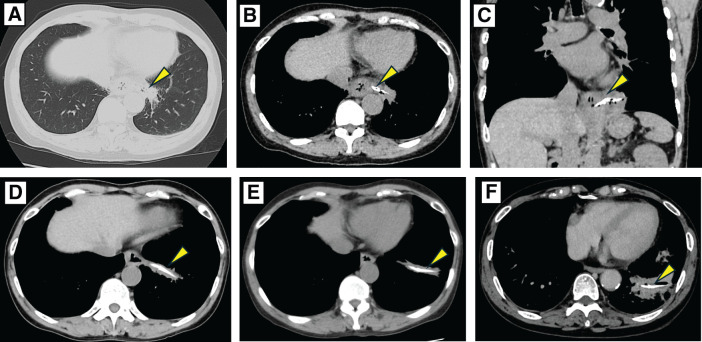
CT images demonstrate peripheral migration of a fish bone from the esophagus into the left lower lobe (LLL). Initial CT at the referring hospital (lung window) shows parahilar consolidation in the LLL (A). Corresponding mediastinal window images reveal a linear high-attenuation foreign body measuring approximately 4 cm, extending from the esophagus toward the LLL (B, C). Follow-up CT at 3 months shows distal migration of the foreign body within the lung parenchyma (D), and further progression toward the periphery is noted on the 1-year follow-up CT (E). Five years after the initial presentation, CT performed at our institution shows the foreign body lodged in the peripheral LLL, surrounded by a small cavity and adjacent consolidation (F). (Arrowheads indicate the foreign body.)
